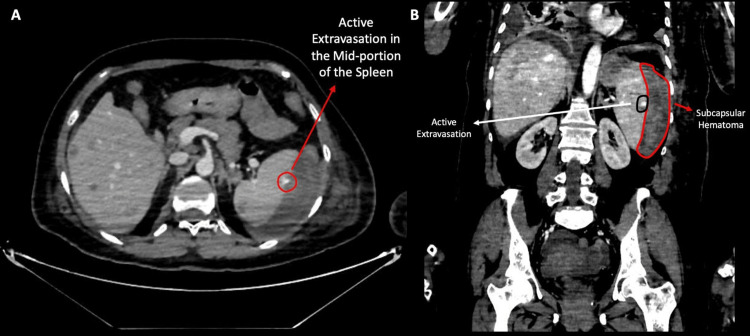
Post-contrast CT images demonstrating active splenic contrast extravasation with associated subcapsular hematoma A) Post-contrast axial CT image demonstrating a hyperdense area consistent with active splenic extravasation B) Post-contrast coronal CT image demonstrating a hyperdense area indicating active splenic extravasation together with a subcapsular hematoma
